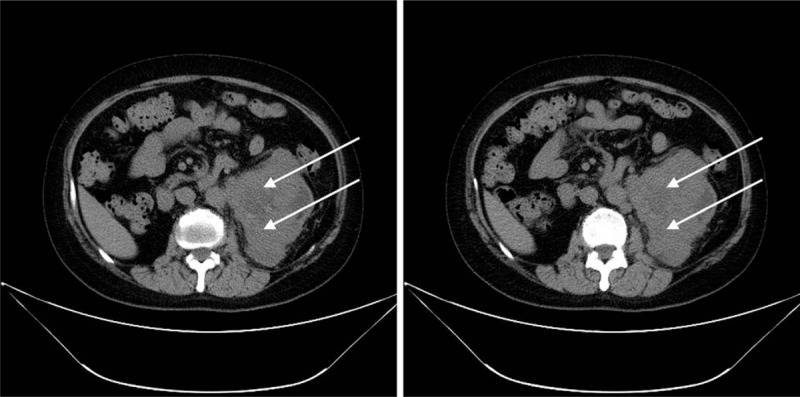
Computed tomography (CT) showed a malignant tumor of left kidney. CT = computed tomography.
